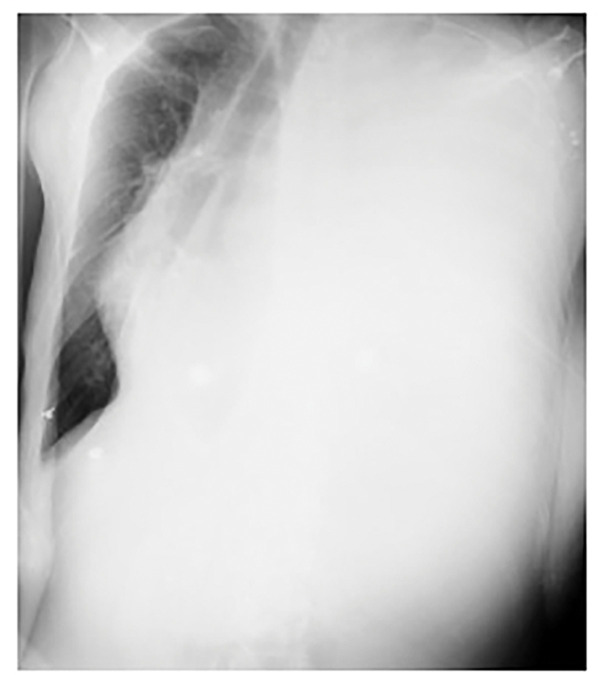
Chest radiograph at hospital admission. A plain chest radiograph at hospital admission showing massive left pleural effusion with mediastinal shift to the right.
